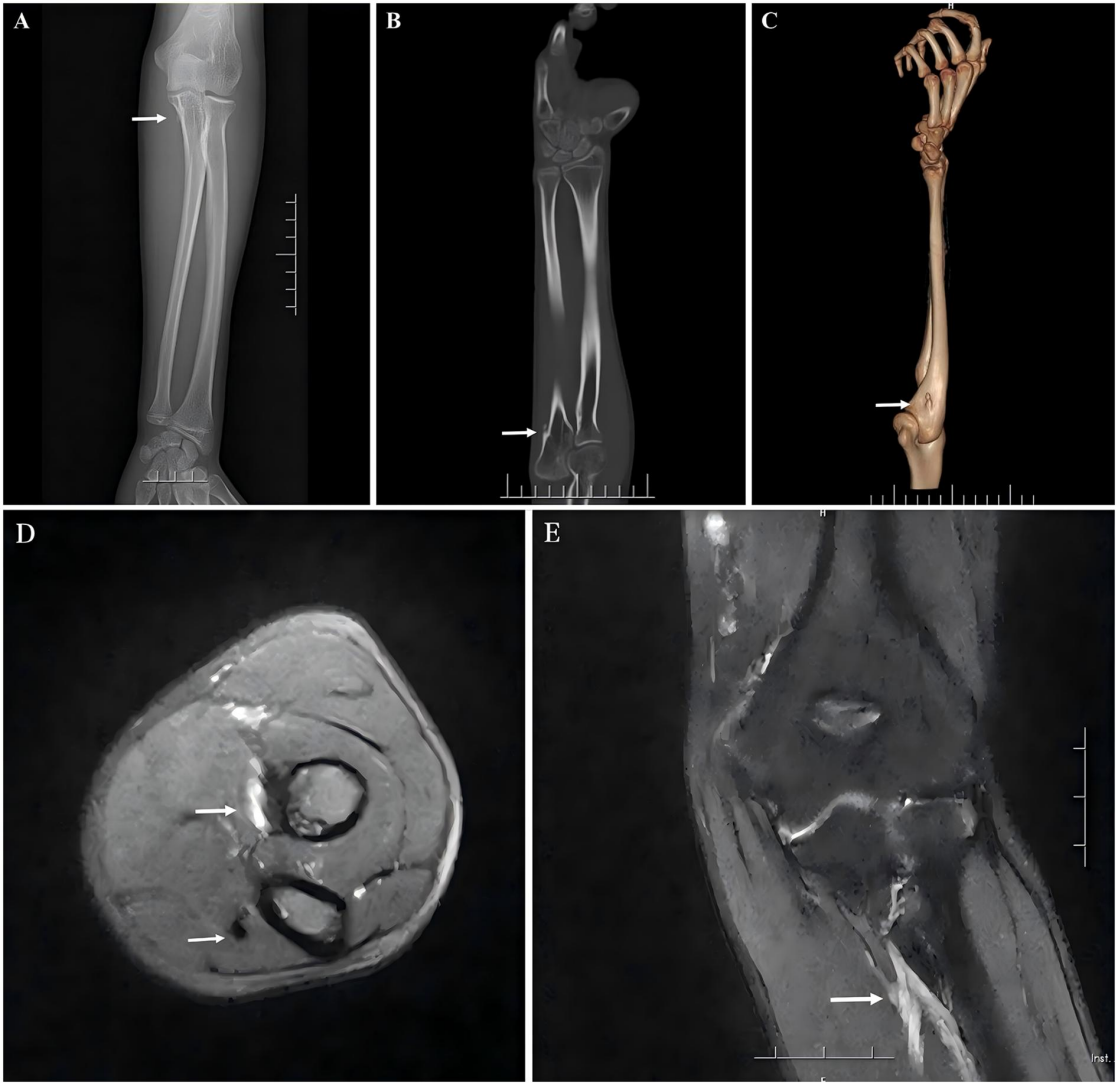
(A,C) x-ray and 3D CT scans reveal a bony prominence on the medial aspect of the proximal ulna (indicated by arrow). (B) The coronal CT scan of the ulna shows that the bony prominence originates from the cortical surface of the ulna, extending distally (indicated by arrow). (D) MRI shows a high-intensity lesion in the deep muscle layer of the anterior proximal forearm and an abnormal fibrous cord in the flexor digitorum profundus. (E) A high-intensity lesion in the deep muscle layer of the anterior proximal forearm.
